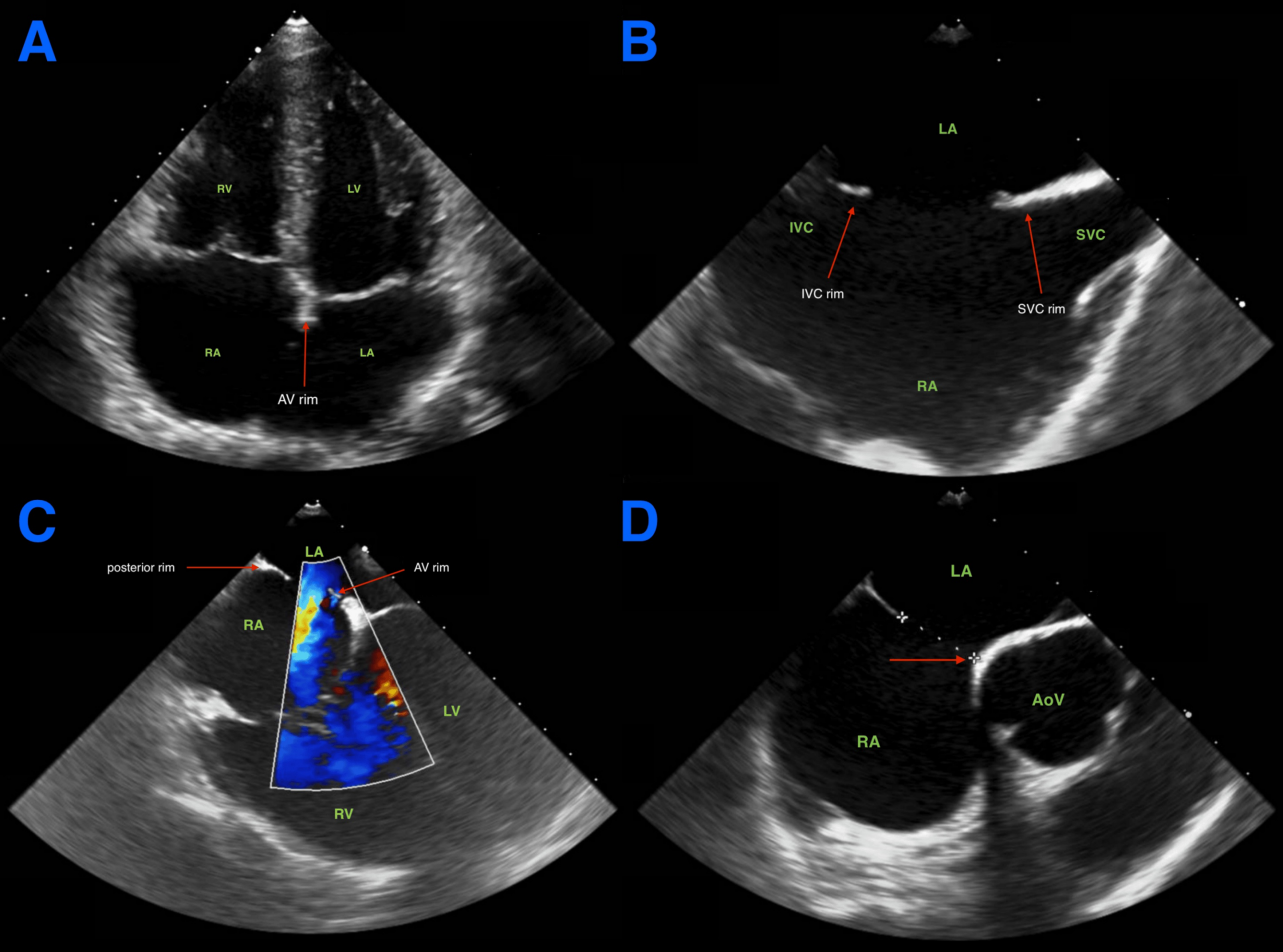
Preoperative echocardiography showing the atrial septal defect rims A: TTE (4-chamber view) showing the atrio-ventricular rim
